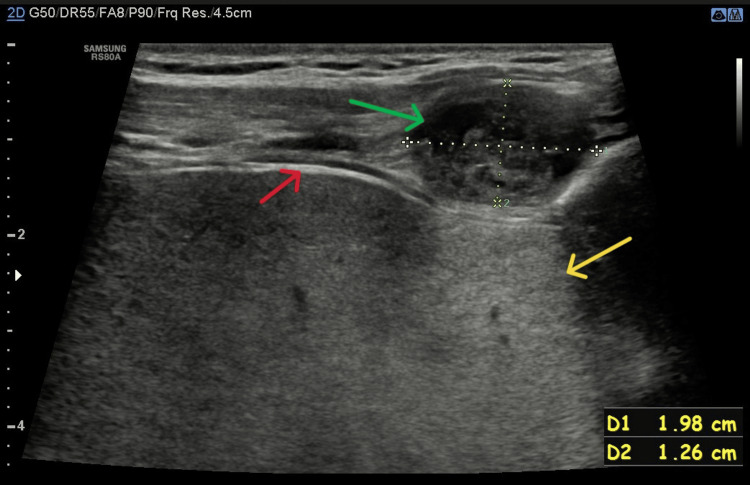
Image from ultrasound examination: lesion with cystic elements (green arrow), visceral pleura (red arrow), and posterior acoustic enhancement (yellow arrow) is presented
